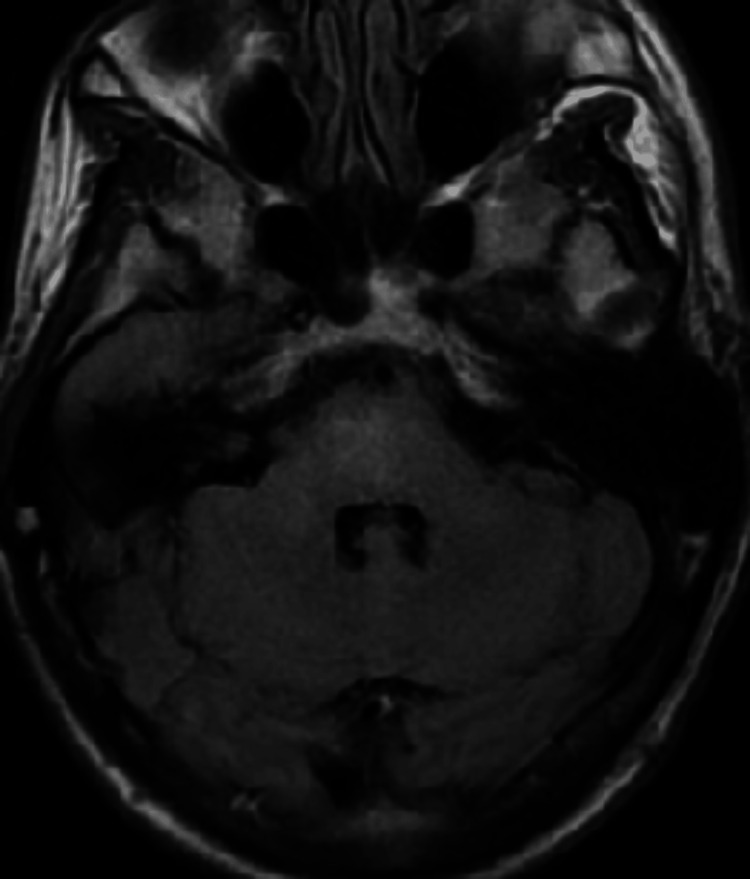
MRI brain axial view of the T2 FLAIR sequence done at week five from the symptom onset.
